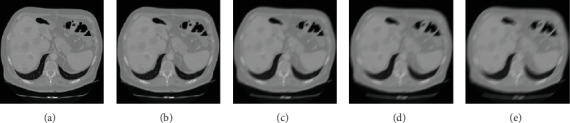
The results of different blurred amplitude of the liver tumour patient. (a) Original CT image. (b) Blurred amplitude of 5. (c) Blurred amplitude of 15. (d) Blurred amplitude of 20. (e) Blurred amplitude of 25.
